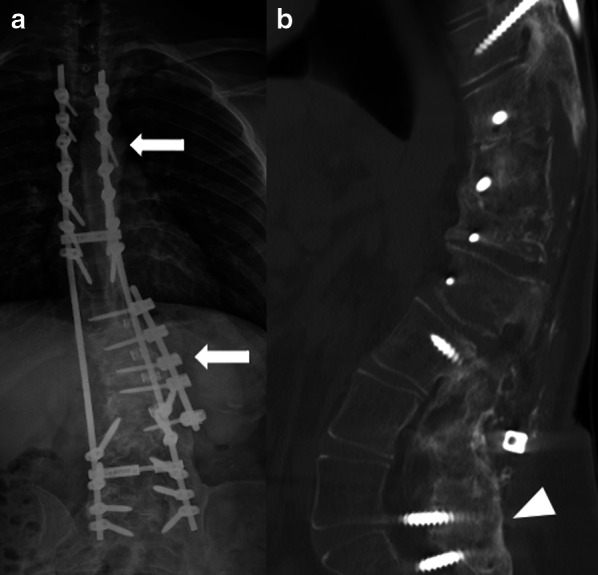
Stabilization construct for deformity correction (scoliosis). Anteroposterior (a) radiograph and sagittal CT reformat in bone algorithm (b) demonstrate a fusion/stabilization procedure using bilateral rods with transpedicular and lateral body screws (arrows) extending through the thoracolumbar spine (from T4-T5 to L5-S1). There is no sign of loosening (such as lucency around the screws) or fracture of components. Note the fusion (arthrodesis) of the posterior elements of the lumbar spine (arrowhead), indicating a successful outcome
